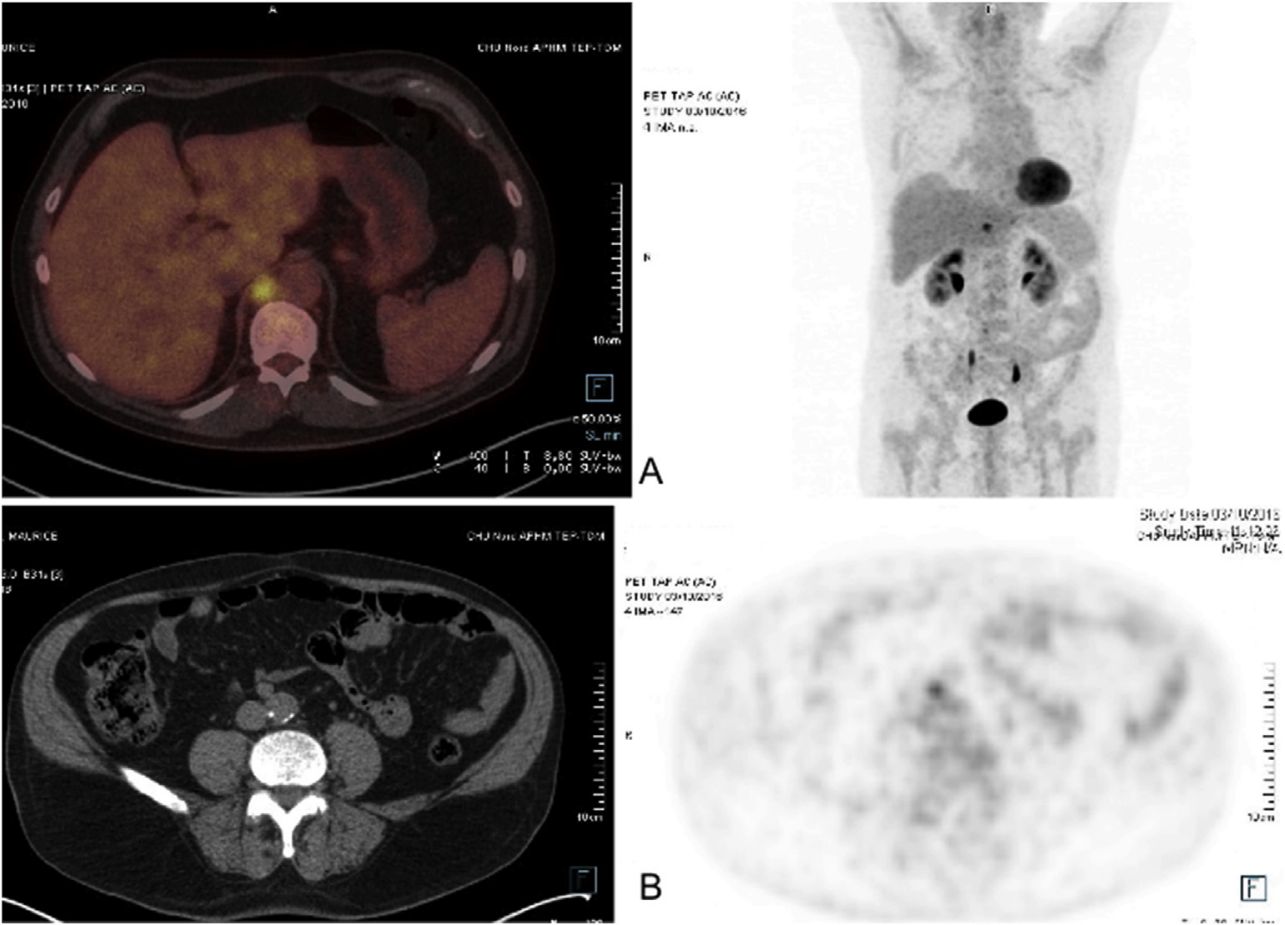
PET-CT at 24 months post-operatively showing: A. lower mediastinal hypermetabolic lymphadenopathy - B. a less hypermetabolic retroperitoneal lymphadenopathy.
